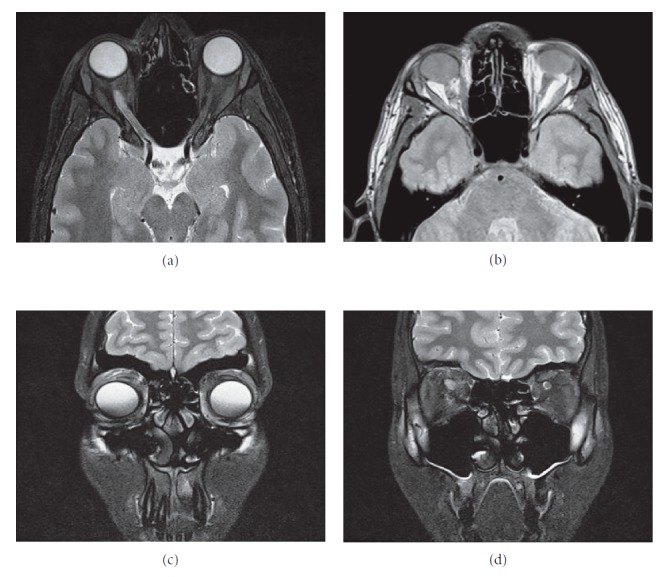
Postoperative MRI scans (3 months). Axial and coronal T1 (a, c) and T2-weighted (b, d) MRI images show normal anatomical conditions with no residual tumor mass.
